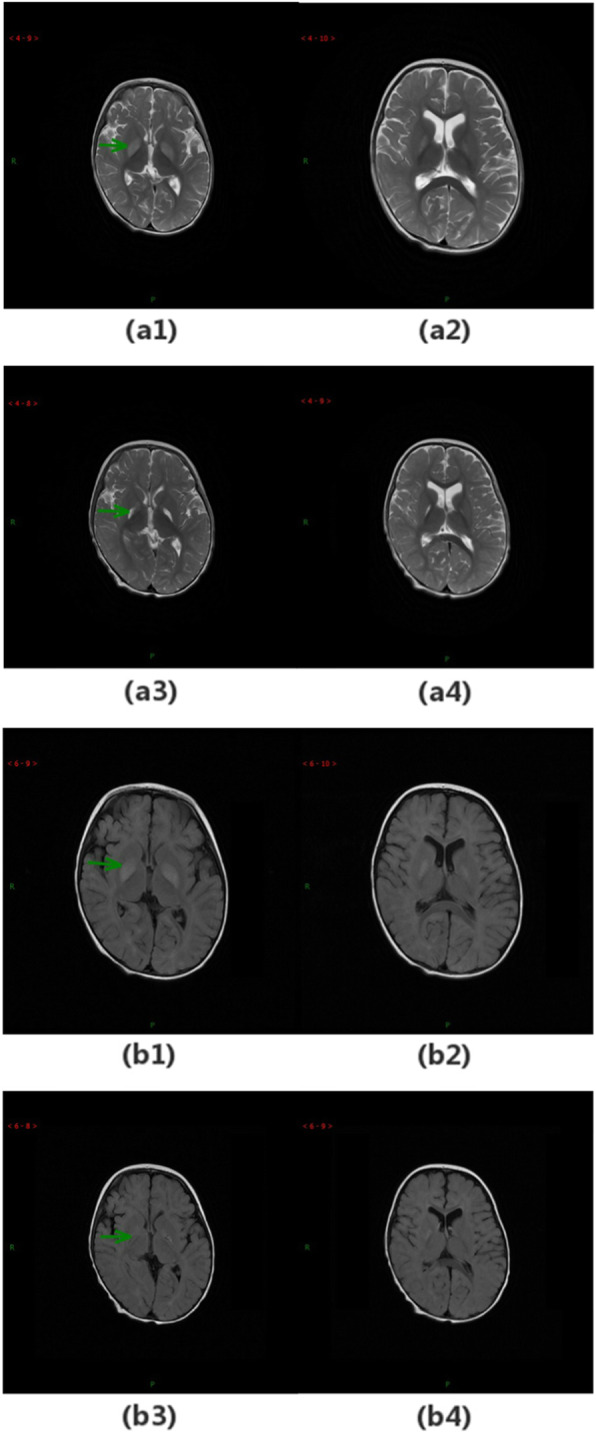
MRI images of patient P5 with green arrows showing abnormal areas. Images (a1–4) and (b1–4) are T2-weighted and T2-FLAIR images, respectively. Images (a1–2) and (b1–2) are the results of the first MRI, which show symmetric abnormal signals of the bilateral basal ganglia. Images (a3–4) and (b3–4) show the results of the second MRI, which were similar to the first MRI
